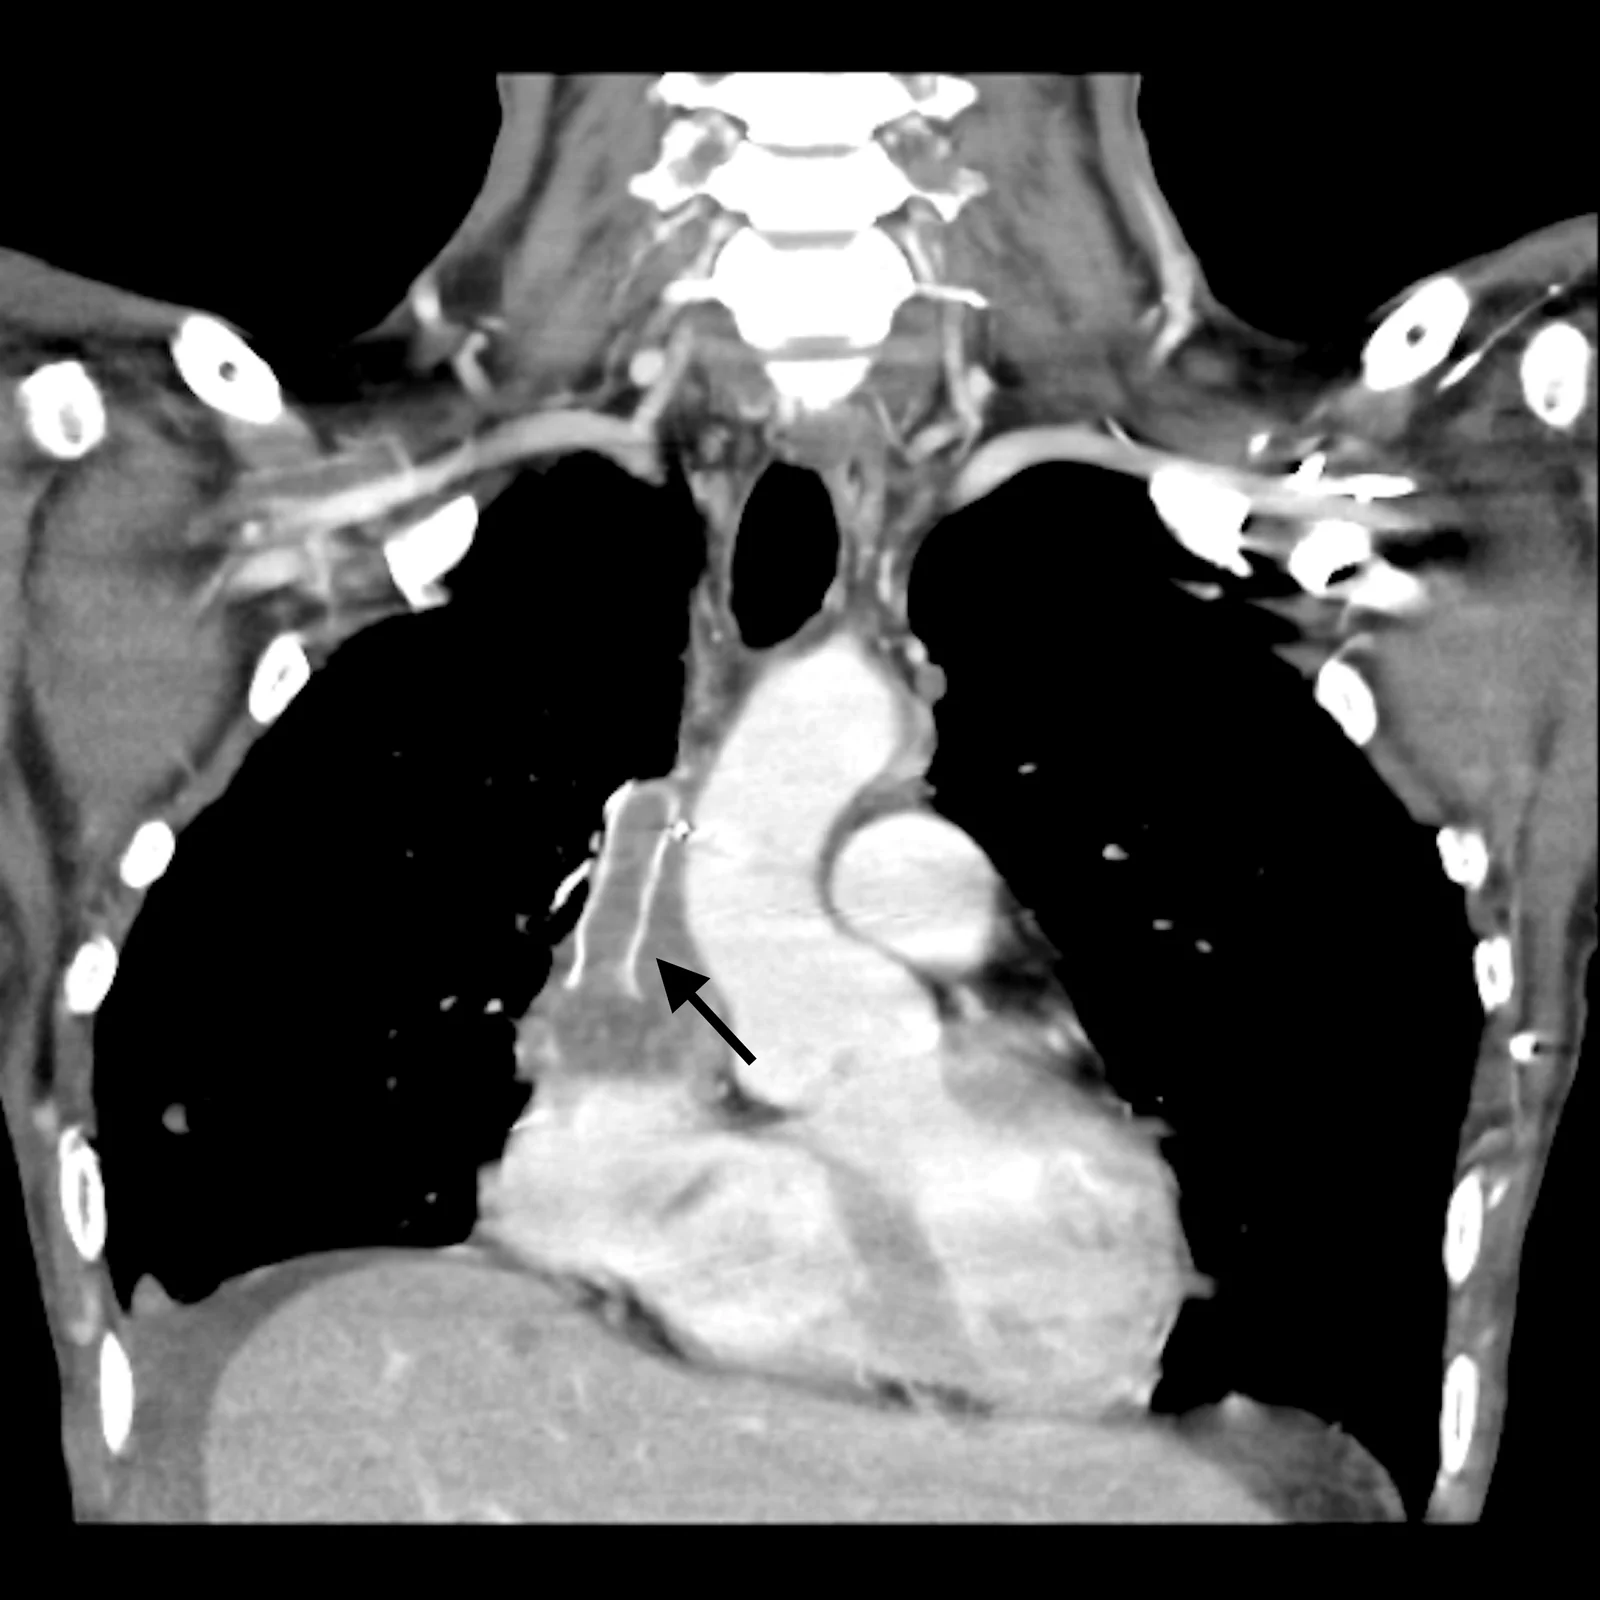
Postoperative contrast-enhanced chest CT sagittal view showing a patent SVC/PTFE graft. The arrow indicates the reconstructed graft segment SVC: superior vena cava; PTFE: polytetrafluoroethylene
